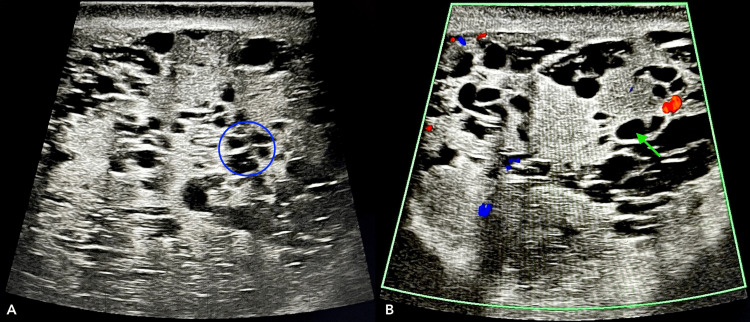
(A) Inguinoscrotal ultrasonography showing multiple cystic tubular structures seen in the testicular mediastinum (blue circle); (B) color Doppler showing these cystic structures (green arrow) taking vascularity on Doppler.
